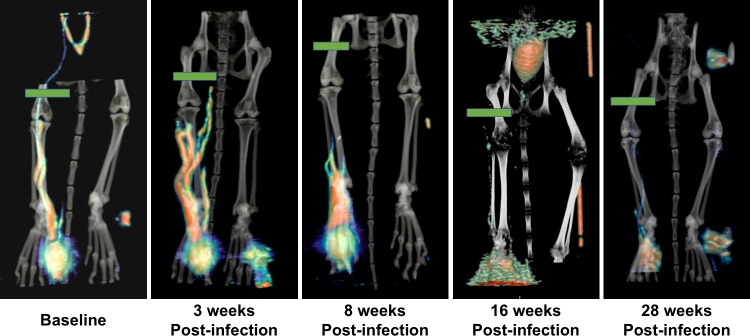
Visualization of lymphatic anatomy using PET imaging. PET and CT images were acquired from ferret F814 pre-infection and 3-, 8-, 20, and 28-weeks post *B*. *malayi* infection. The portrayed maximum intensity projection (MIP) images were constructed by collapsing data from 60 individual PET files (each consisting of a one-minute scan) into a single static frame. The resulting PET image was aligned to the corresponding CT image. Green bars show the designated Region-of-Interest used for kinetic analysis of tracer flow depicted in Fig 8.
